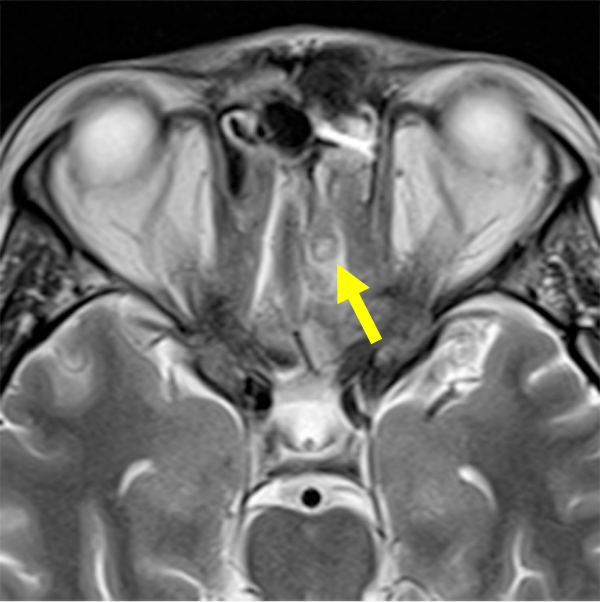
Magnetic Resonance T2-weighted Image of the Brain (Axial Plane). Note the ring-like abscess with perifocal edema (arrow).
